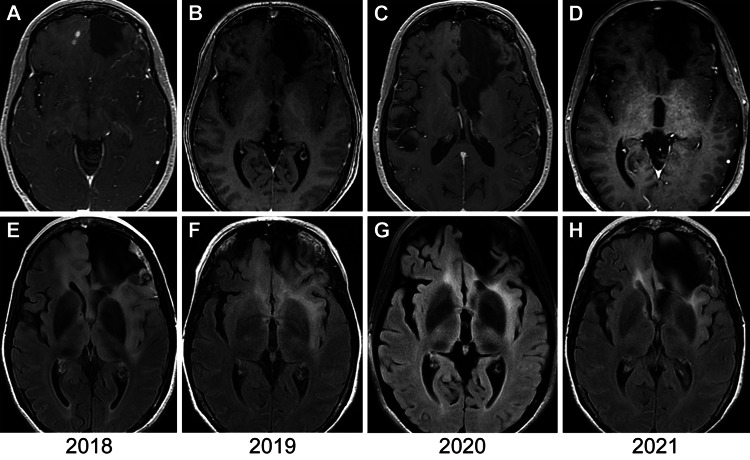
MRIs for patient 3 Axial magnetic resonance imaging (MRI) for patient 3 showing multiple enhancing nodules at the time of recurrence (2018) within the right frontal lobe (A). Annual follow-up T1-enhanced axial MRIs from 2019 (B), 2020 (C), and 2021 (D), obtained during 36 months of continuous ketogenic metabolic therapy, show that the patient experienced complete resolution of tumor enhancement. The patient demonstrated increased fluid-attenuated inversion recovery (FLAIR) signal intensity at the time of recurrence (2018) (E), with progressive improvement in FLAIR signal intensity and distribution in 2019 (F), 2020 (G), and 2021 (H). Used with permission from Barrow Neurological Institute, Phoenix, Arizona.
